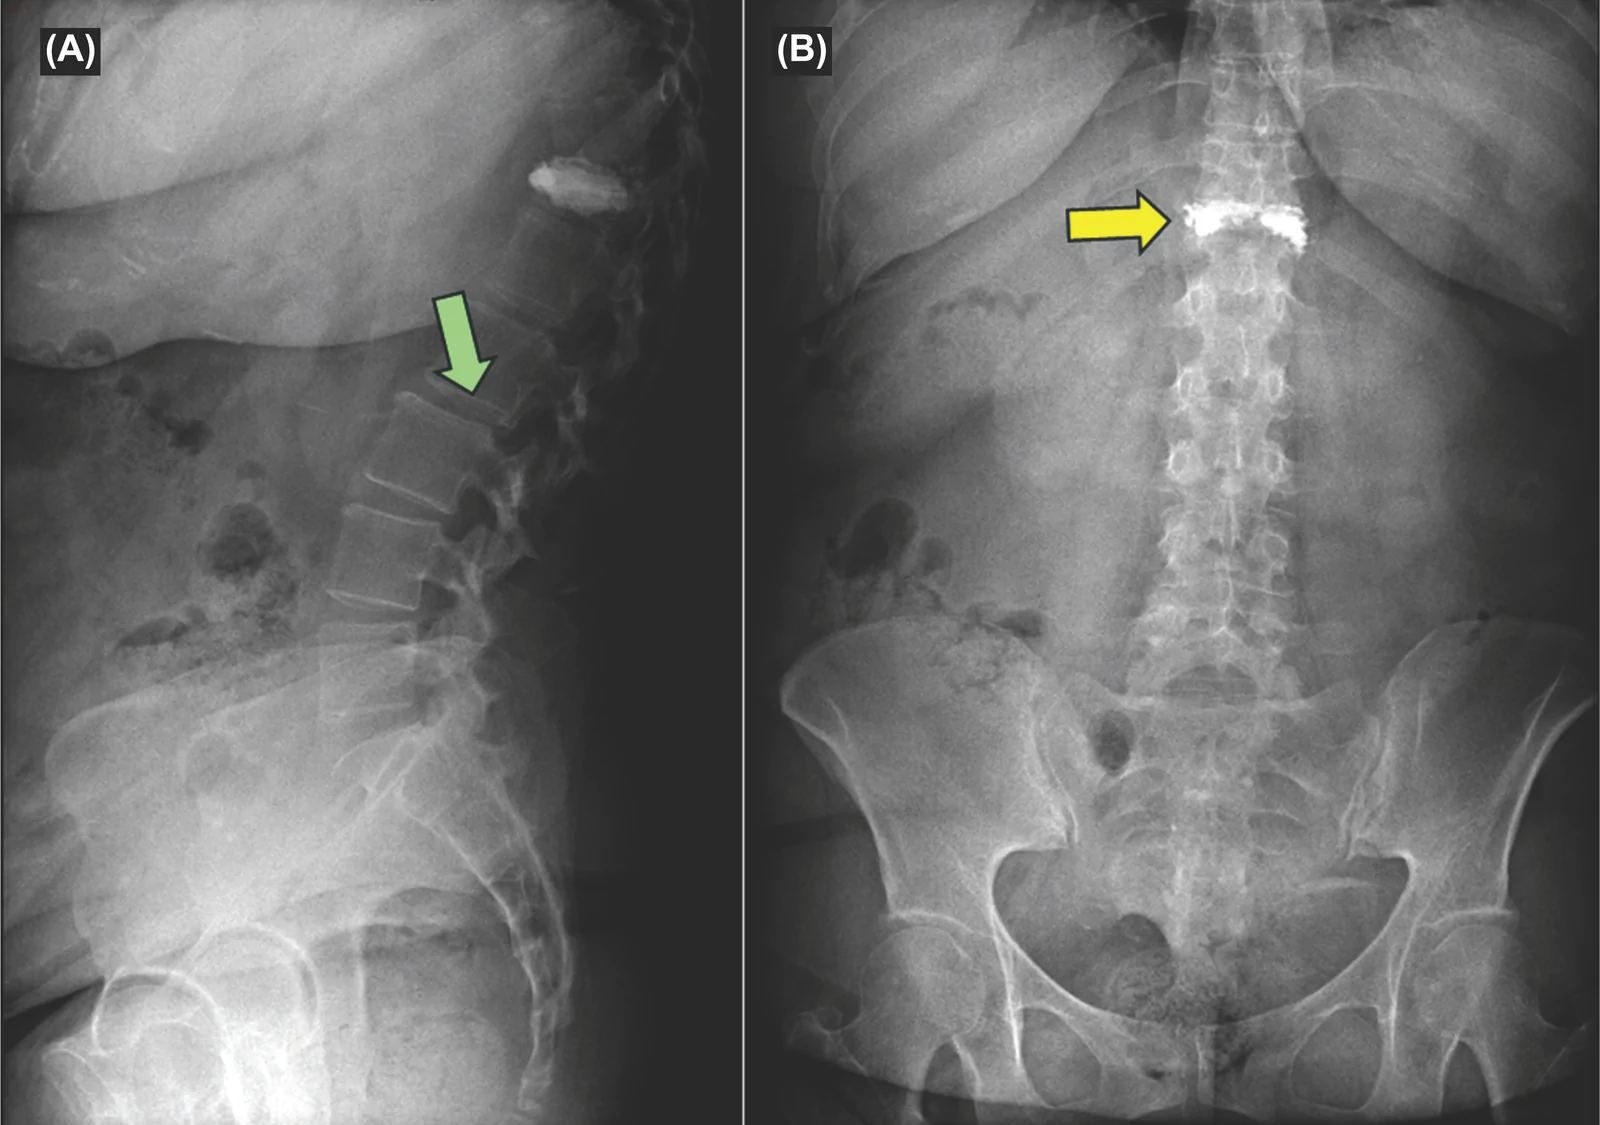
Lumbar spine radiographs [AP (A), lateral (B)]. Reduced T12 vertebral height with hyperdense vertebroplasty cement (yellow arrow) extending toward the left T12 neural foramen; mild subchondral sclerosis and early anterior osteophytes. L2 on L3 posterior displacement <25% (Meyerding grade I, green arrow). Increased thoracic kyphosis. Facet joints and posterior elements are preserved. Abbreviation: AP, anteroposterior.
